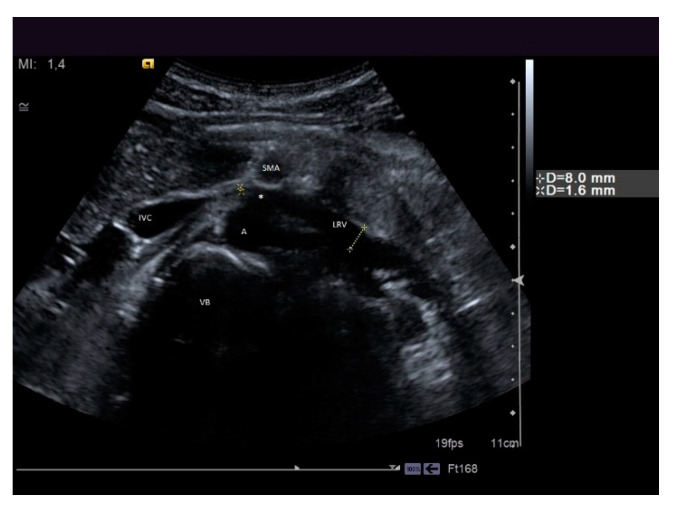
A patient suffering from flank pain, varicocele, and hematuria evaluated at the ultrasound (US) laboratory of the Nephrology and Dialysis Unit. The axial grayscale sonogram demonstrates the anatomical relationships between the left renal vein, aorta, and superior mesenteric artery, with stenosis at the aorto-mesenteric angle (beck sign) and dilation of the hilar section of the left renal vein with a ratio of nutcracker phenomenon. A: aorta, SMA: superior mesenteric artery, LRV: left renal vein, IVC: inferior vena cava, VB: vertebral body, white asterisk: beck sign.
